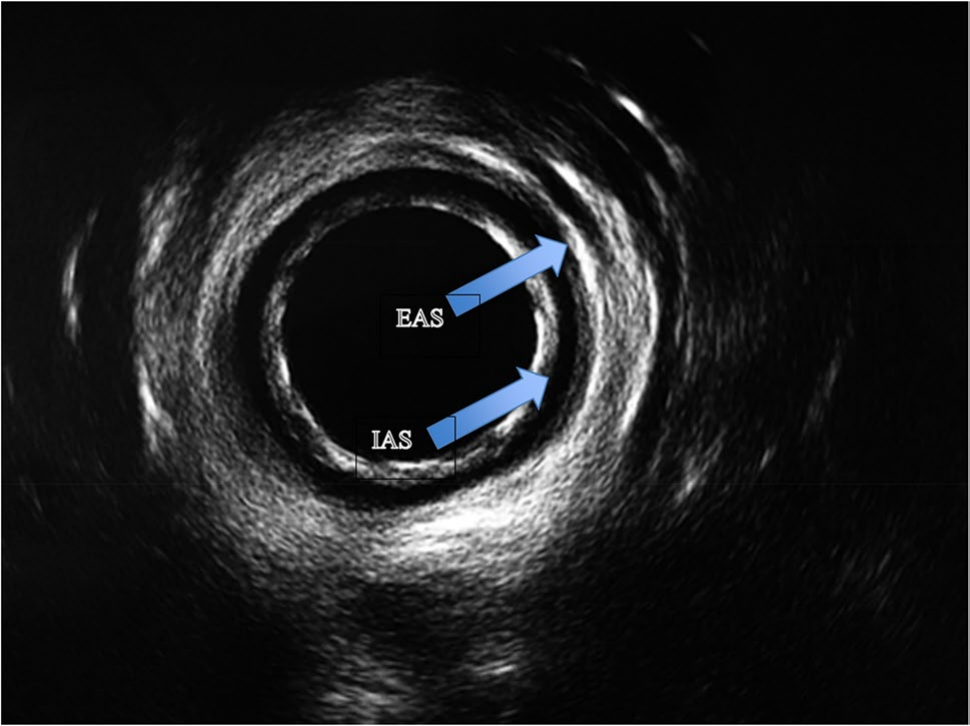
Endoanal ultrasound images showing normal appearance of the internal (IAS) and external (EAS) anal sphincters [47]. *Source:* Reprinted by permission from Springer Nature
